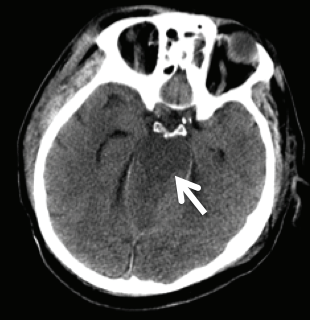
Cranial CT image obtained on the second hospital day at our institution. This axial cut demonstrates extensive brain stem infarction (white arrow) attributed to bilateral vertebral artery occlusion.
